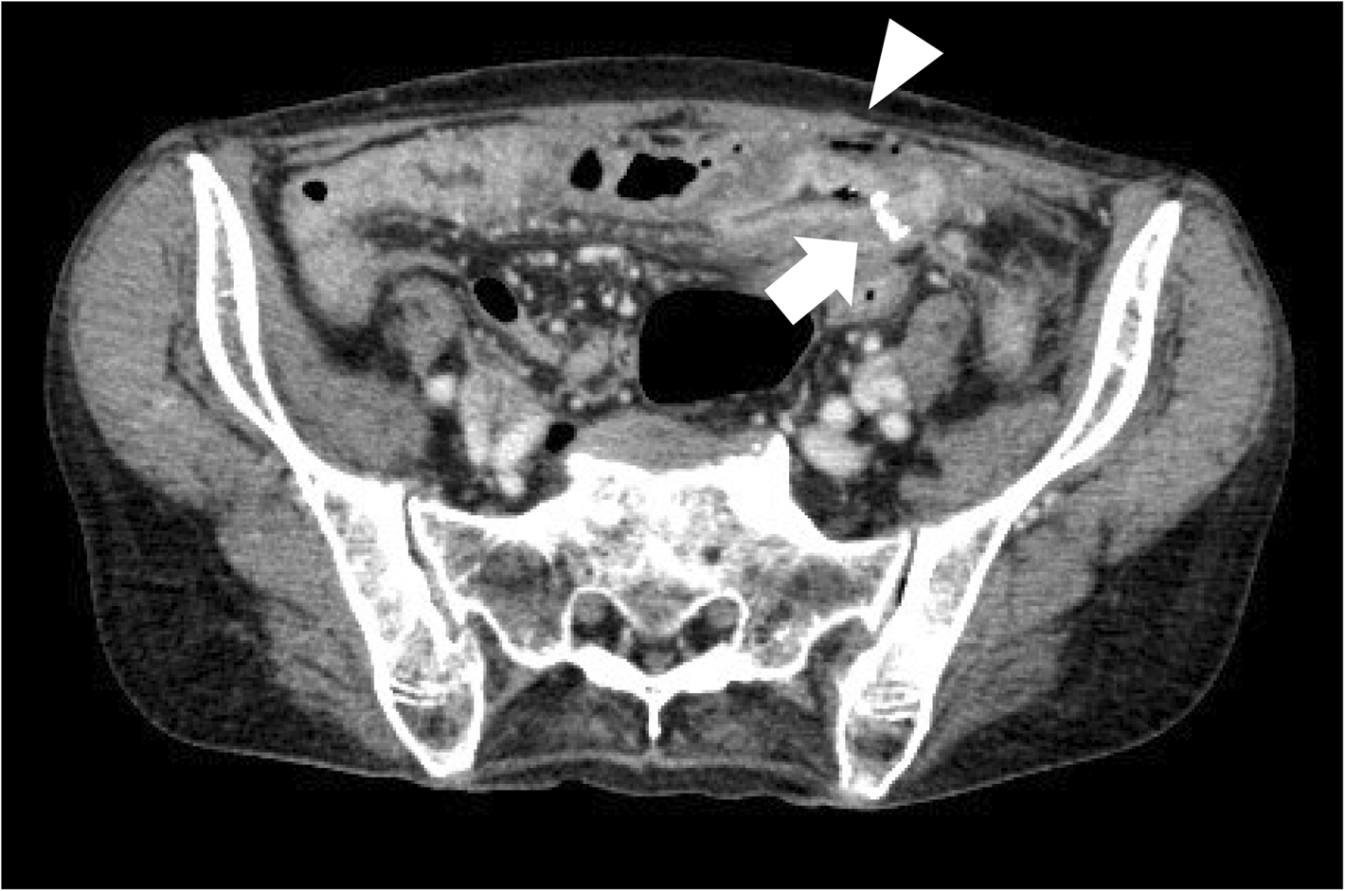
Computed tomography revealed intestinal edema at the duodenojejunostomy site where linear staplers were used (arrow). Free intraabdominal air was observed near the anastomosis site (arrowhead)
